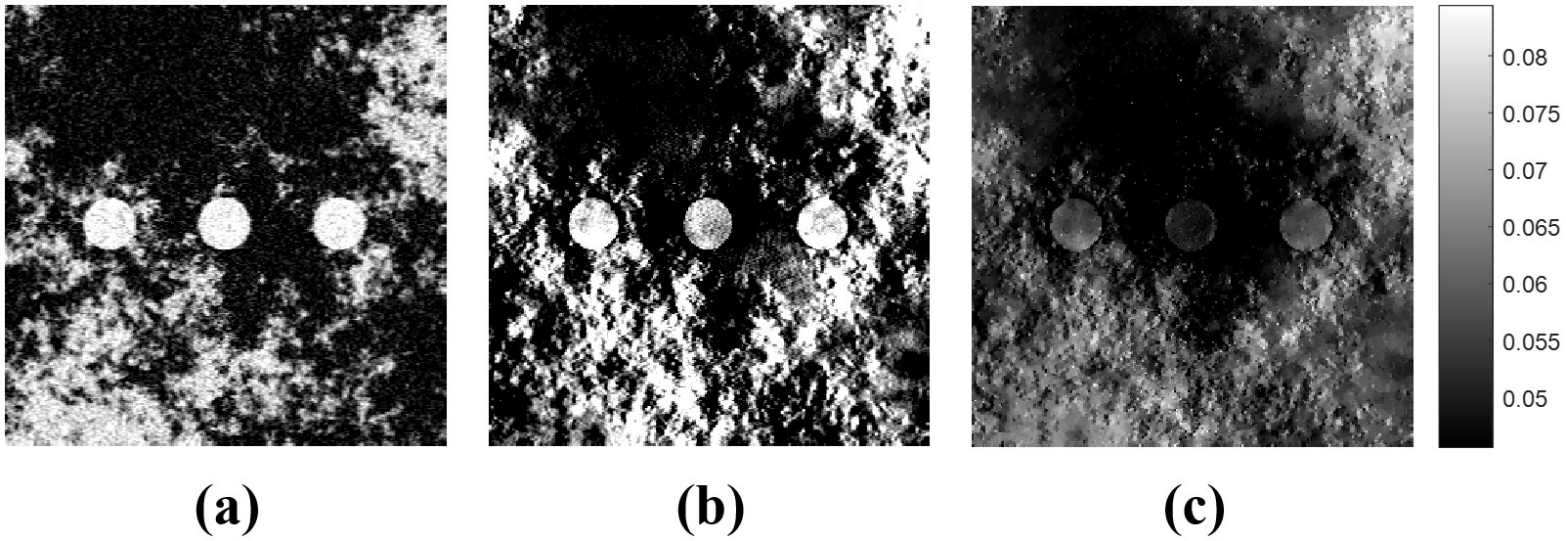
Limitation of the conventional DBT reconstruction algorithm. (a) CBCT images, (b) DBT images reconstructed using FDK, and (c) DBT images reconstructed using GP-BB. The display window is [0.0456 0.0844] *cm*^−1^.
